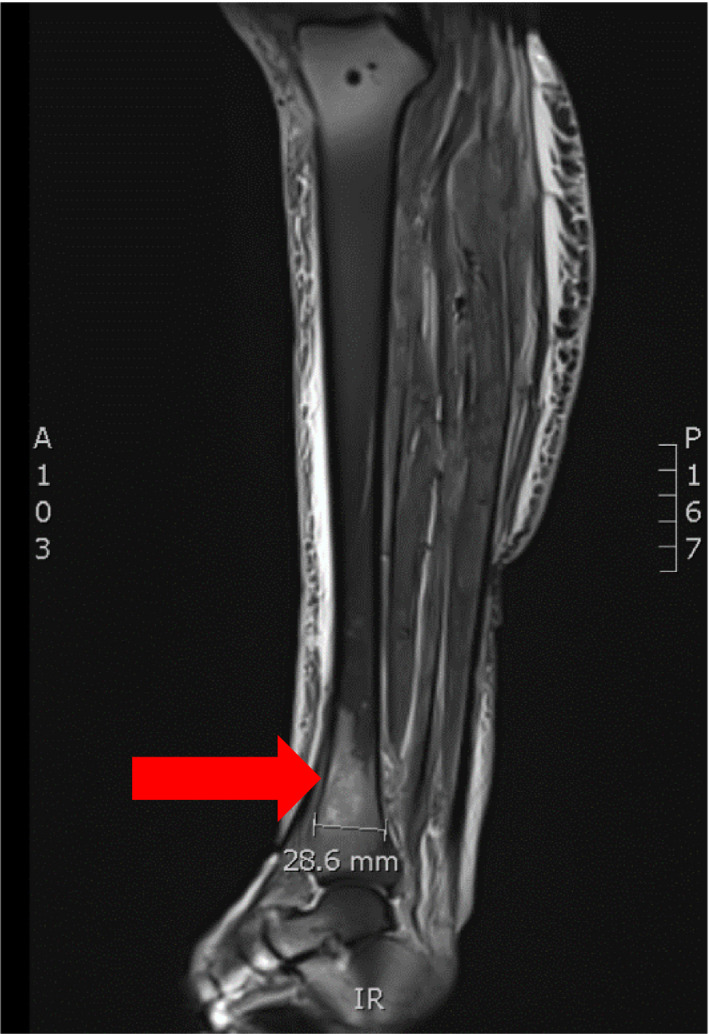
MRI right tibia‐fibula, sagittal image, showing lesion within the distal tibia with areas of nodular abnormal signal noted throughout the remainder of the tibia
